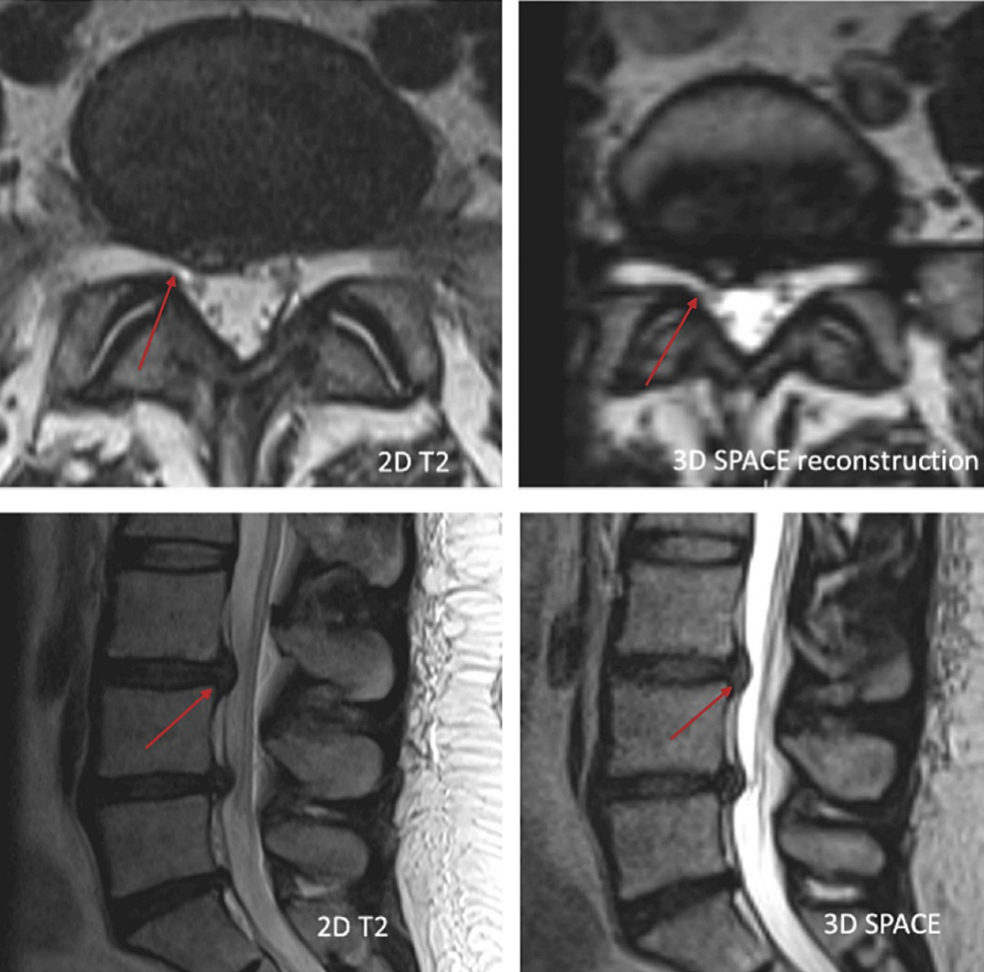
Comparison of 2D MRI and 3D SPACE axial (top row) and sagittal lumbar spine MRI of the same patient. Although higher in-plane resolution of 2D sequences gives crisper images, the presence and severity of spinal canal stenosis can be determined as obviously on SPACE as on the 2D sequences 2D: Two-dimensional; MRI: magnetic resonance imaging; 3D: three-dimensional; SPACE: Sampling Perfection with Application-optimized Contrasts using different flip angle Evolution
